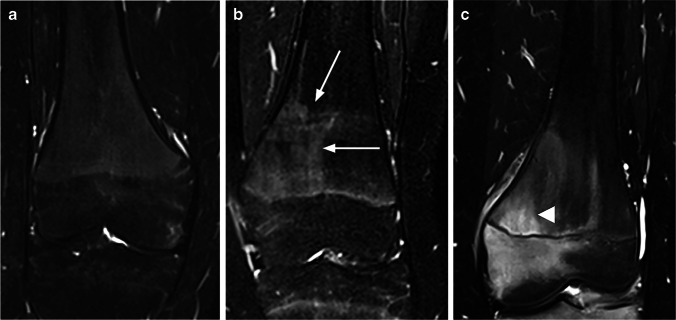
Coronal water-only Dixon T2-weighted magnetic resonance images in (a) a 16-year-old girl with only background signal intensity in the femoral metaphysis, (b) a 15-year-old girl with an area of high signal intensity grade 1 in the metaphysis of the femur (*arrows*) and (c) a 17-year-old boy with an area of high signal intensity grade 2 in the metaphysis of the femur (*arrowhead*)
